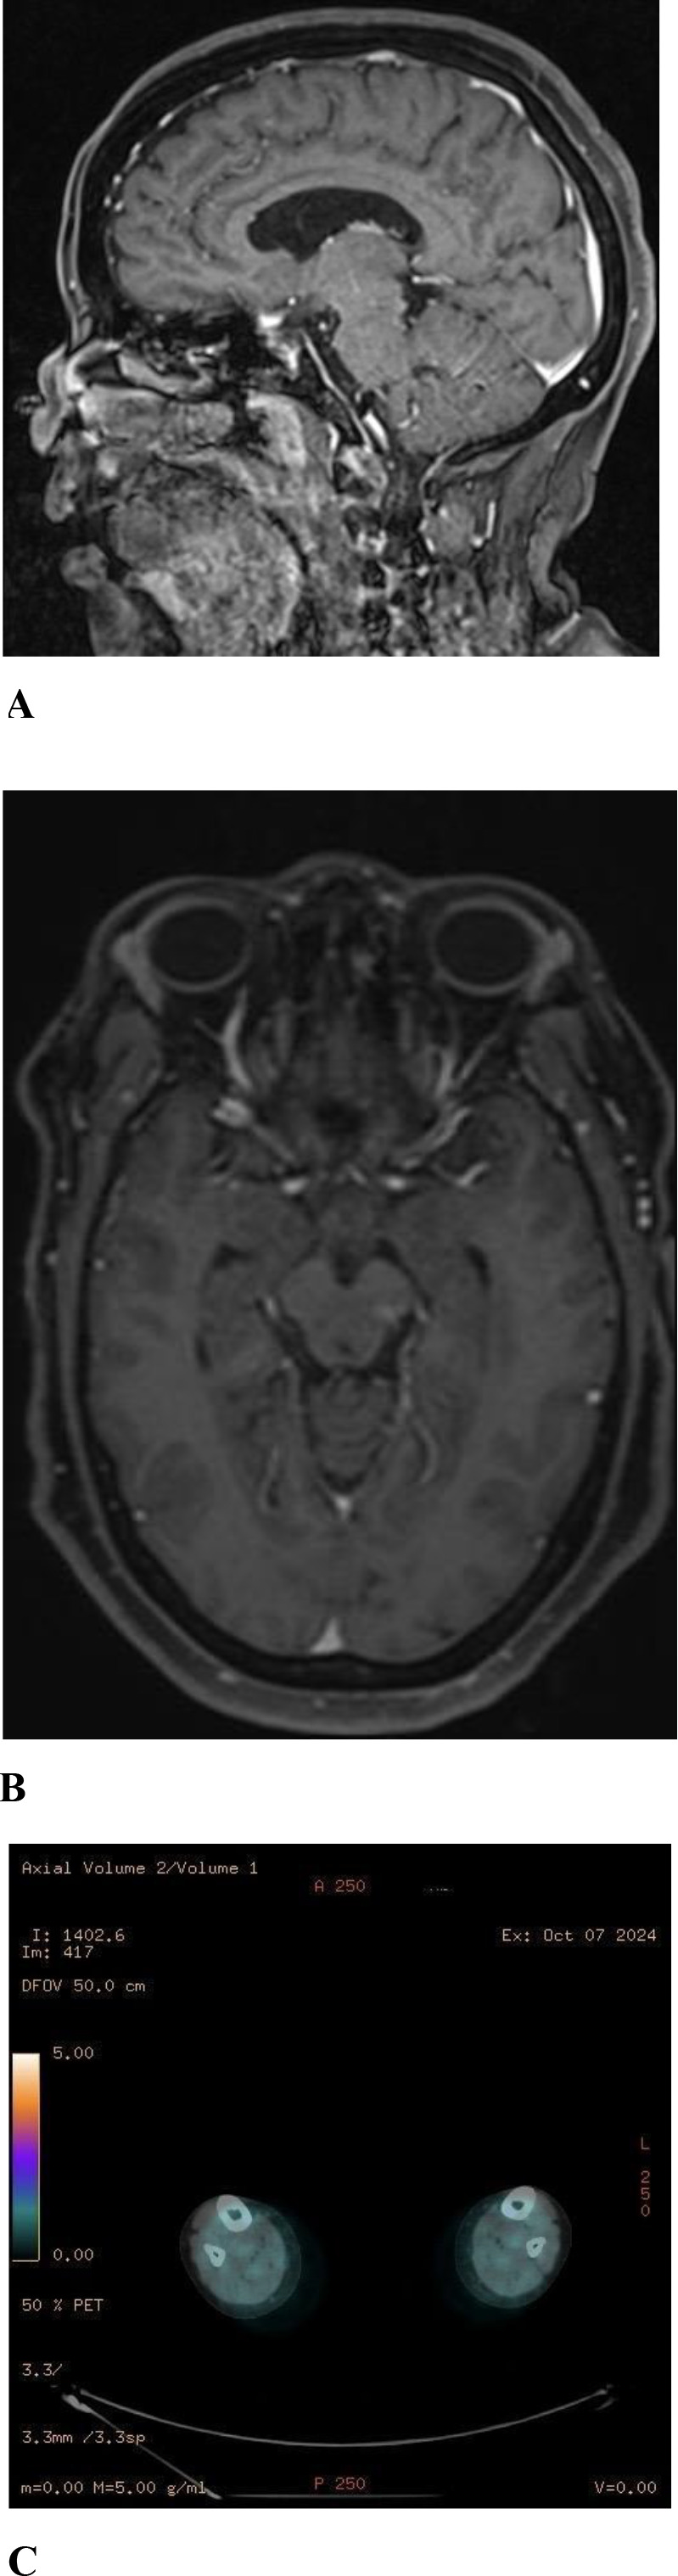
Post-treatment Radiologic images (2024): Axial (B) and sagittal (A) post-contrast T1-weighted Brain MRI showing complete resolution of the previously seen suprasellar enhancing lesion. No abnormal enhancement seen in the tuber cinereum, hypothalamus, lamina terminalis or interpeduncular cistern. Axial (C) FDG PET/CT showing Interval metabolic improvement of the tibial hypermetabolic heterogeneous uptake.
